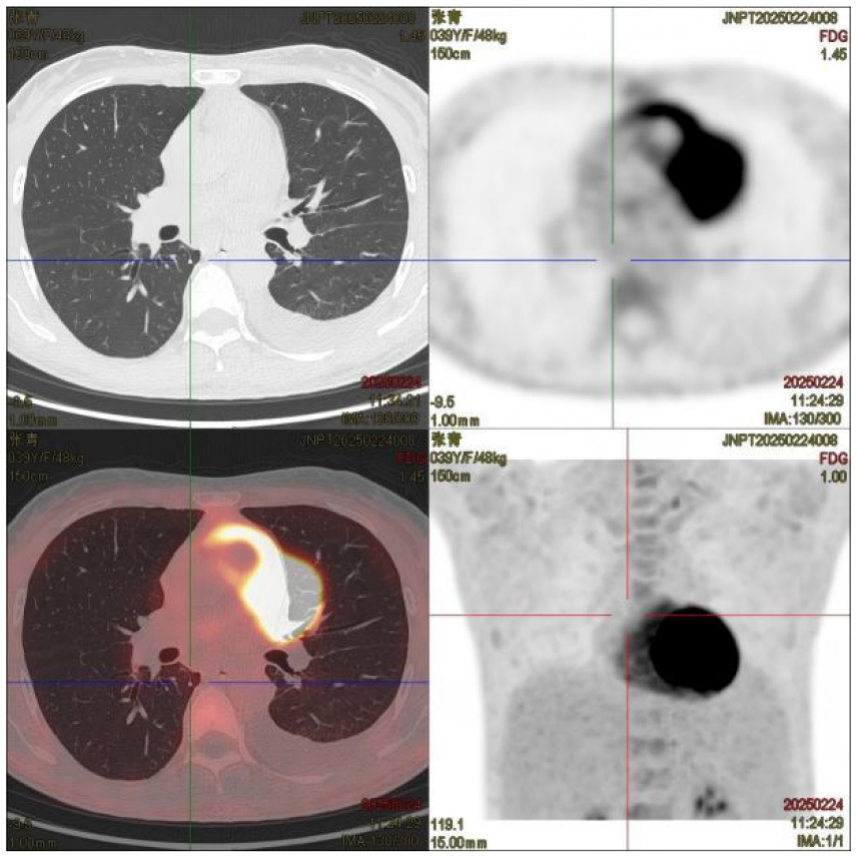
(E) PET-CT at admission (25 February 2025), cervical vertebrae	(F) Abdominal CT after treatment (27 April 2025)
